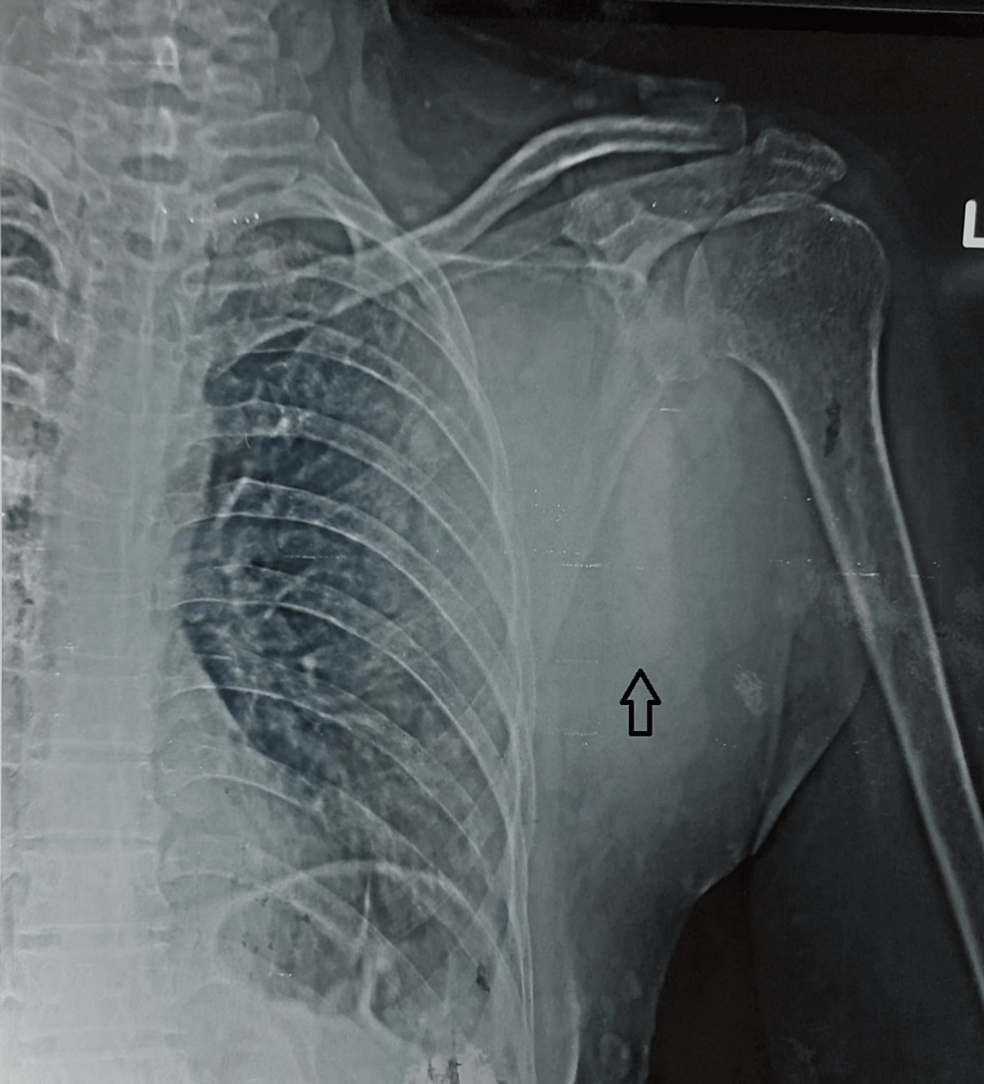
X-ray chest showing large lobulated soft tissue seen within the subcutaneous tissue along the left lateral scapular margins
